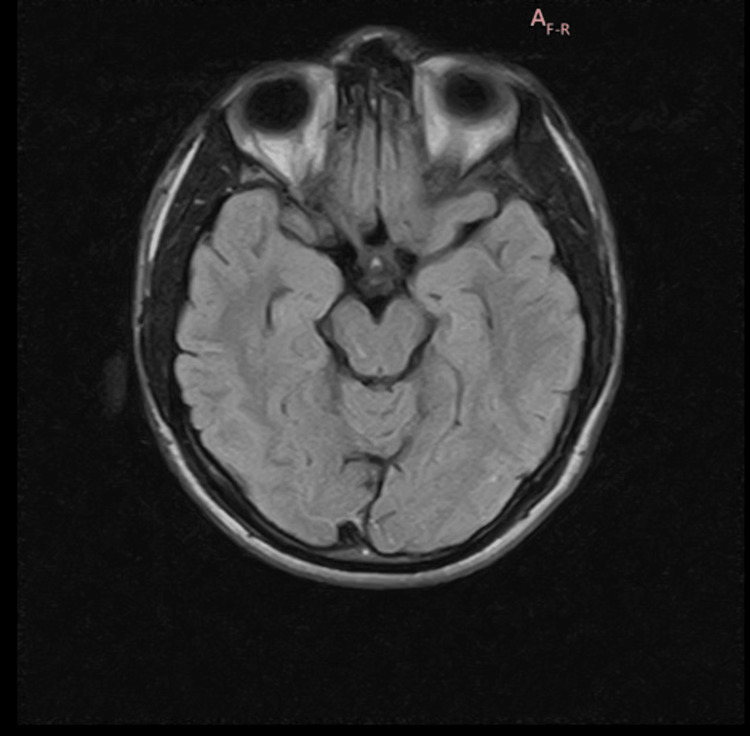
T2 axial FLAIR sequence of MRI brain showing no abnormalities
